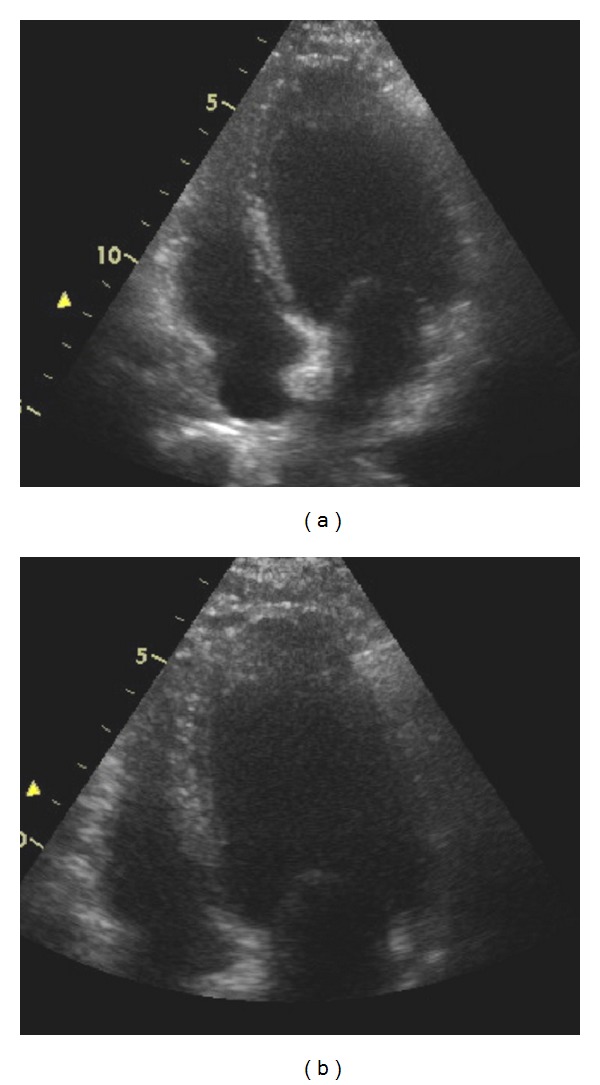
Echocardiogram showed a severely dilated left ventricular apex with associated thrombus.
